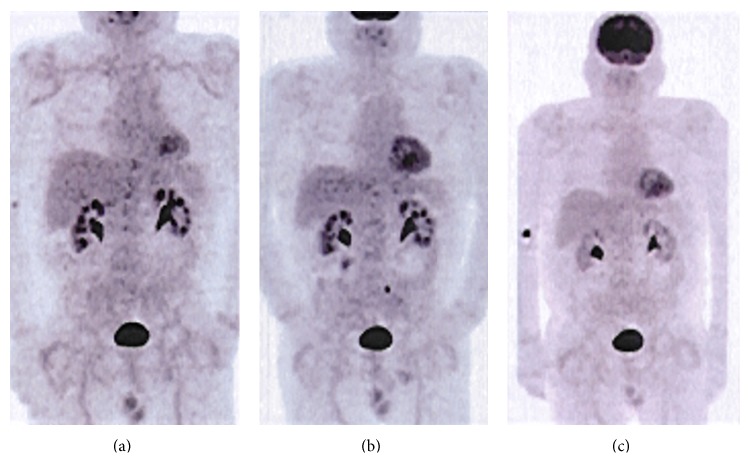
Sequential PET scan images. (a) One-year follow-up: medium intensity uptake of 18F-FDG at the subclavian, carotid, and femoral arteries. (b) Two-year follow-up: medium intensity uptake of 18F-FDG at the femoral and right external iliac. (c) Three-year follow-up: absence of 18F-FDG uptake. PET: positron emission tomography. 18F-FDG: 18F-fluorodeoxyglucose.
